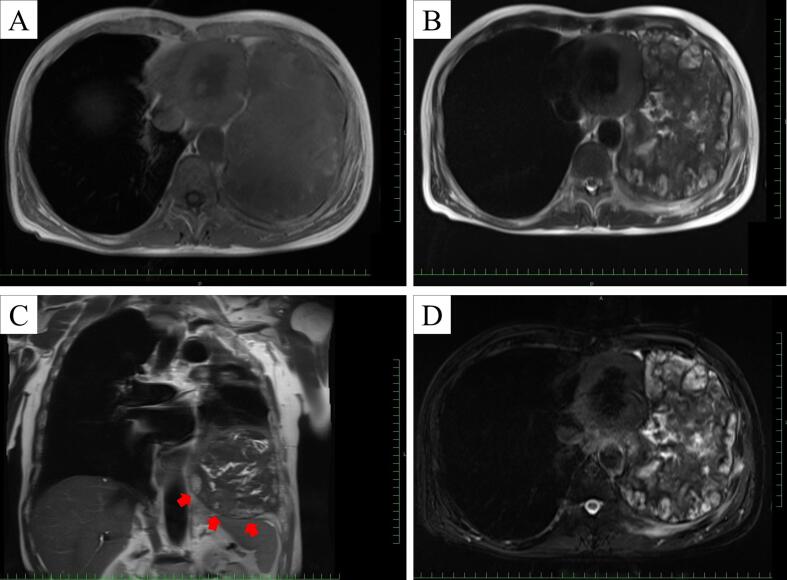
(A) T1-weighted magnetic resonance imaging shows a mass with predominantly low signal intensity. (B) Mosaic of various signal intensities on T2-weighted images. (C) Thick pseudocapsule (red arrows) with low intensity on T2-weighted images. (D) High signal intensity areas on T2-weighted images were not suppressed on fat-suppressed T2-weighted images. (For interpretation of the references to colour in this figure legend, the reader is referred to the web version of this article.)
